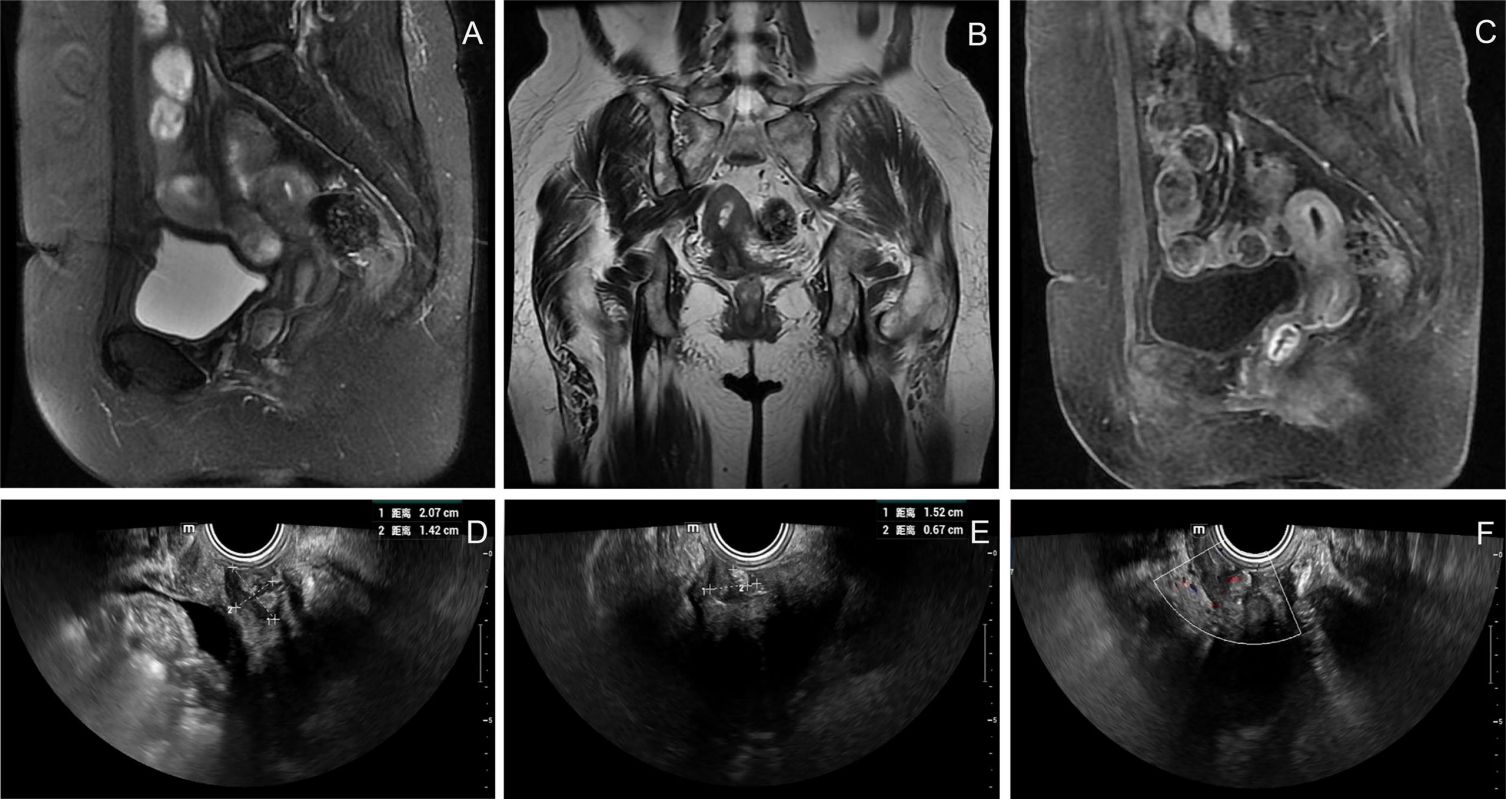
Imaging and ultrasound findings of the patient. (A–C) Pelvic enhancement MR: an oval equal T1 mixed T2 signal shadow was observed on the anterior wall of the upper segment of the vagina, approximately 1.7 cm × 1.4 cm × 1.9 cm, with obvious ring reinforcement; (D–F) Pelvic ultrasound: a 2.1 cm × 1.5 cm × 1.4 cm mass was observed on the anterior wall of the vagina, with a fuzzy boundary and a hypoechoic inside with multiple strong echoes. CDFI could detect blood flow signals.
